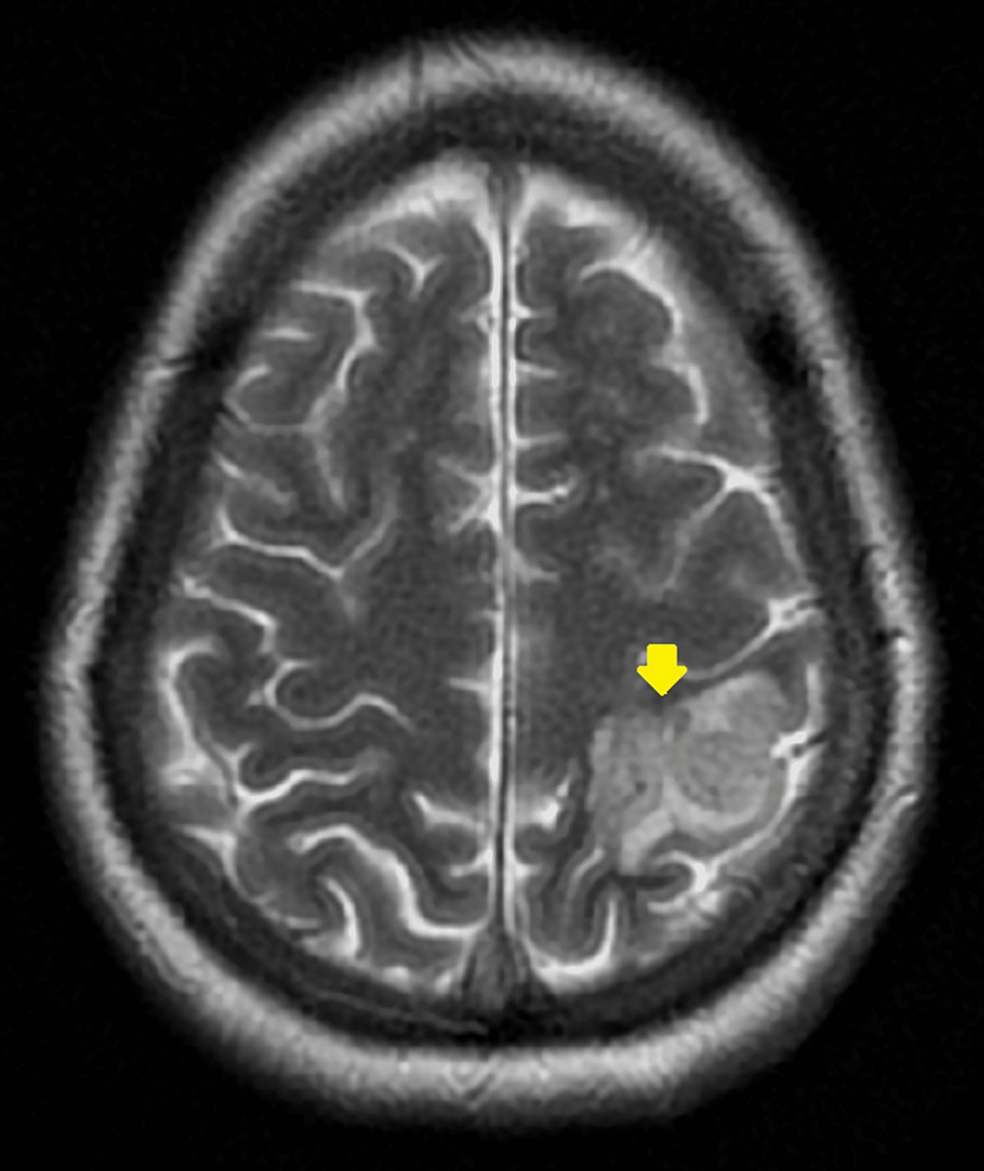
Axial view of T2 weighted MRI depicting hyperintensity in left parietal lobe MRI, magnetic resonance imaging
